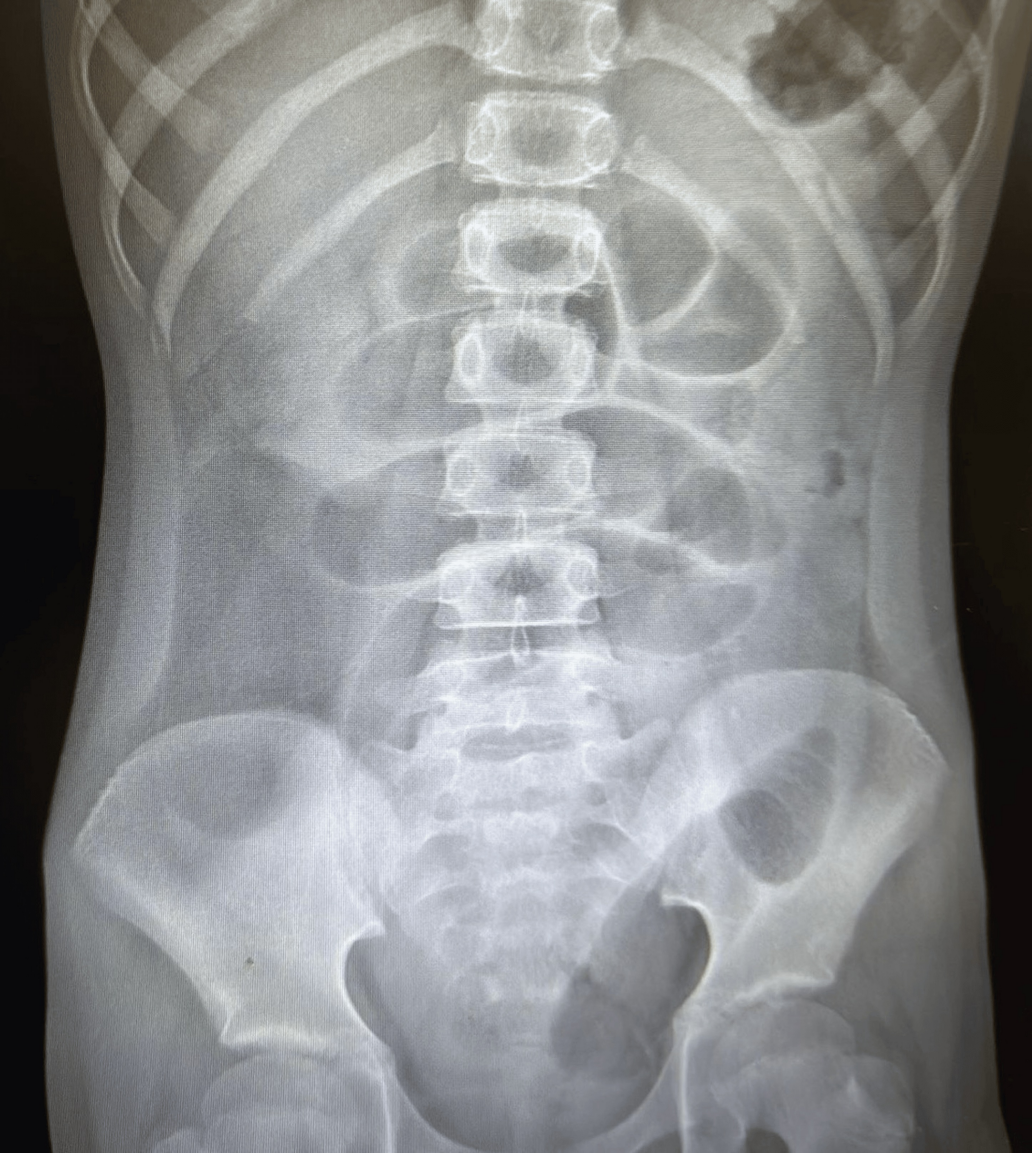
Abdominal X-ray showing multiple air-fluid levels.
